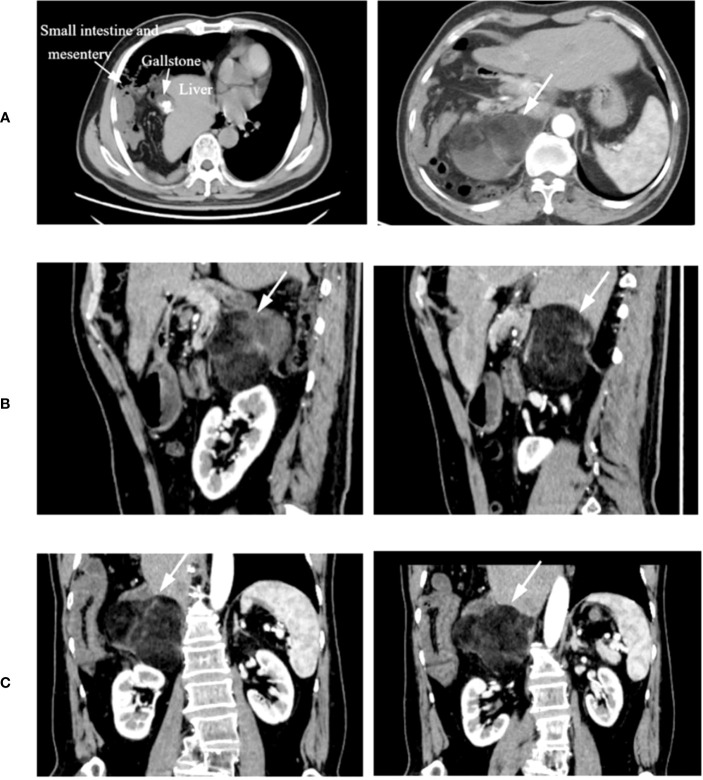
Representative CT images. (A) Cross-sectional CT showing part of the liver and small intestine and mesentery herniated into the thoracic cavity. (B, C) CT sagittal and coronal scans, with the tumor location indicated by the finger.
